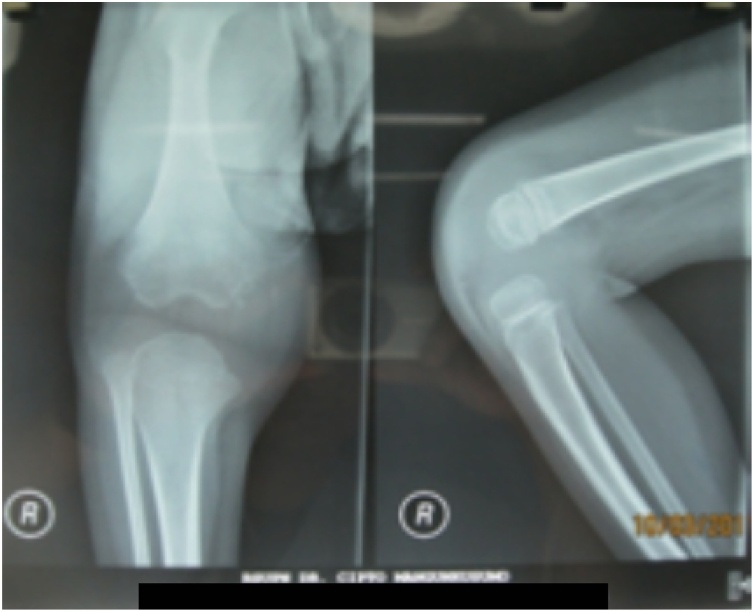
Plain Radiography of the Right Knee Joint. The findings were irregularity of the epiphysis with widening of joint space and soft tissue swelling.
